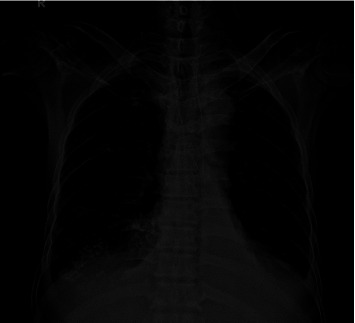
Stent position and expansion in chest X-ray.
